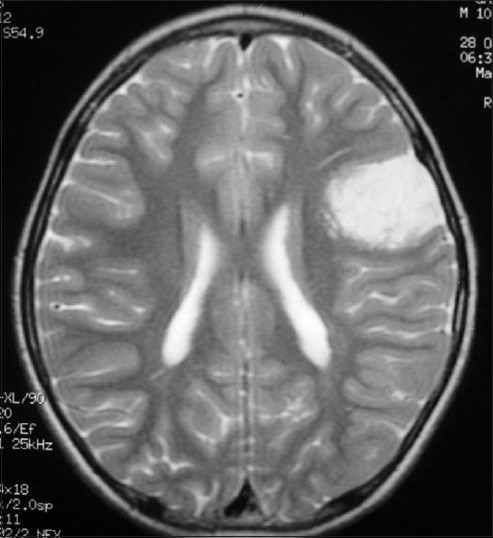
Brain MRI scan shows a large superficial and temporal mass
